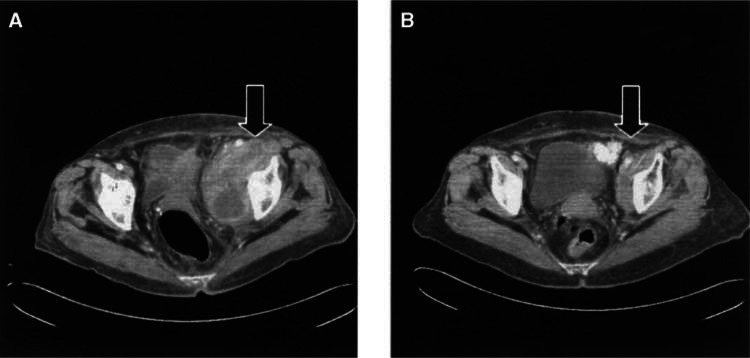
(A) Pelvic CT prior to initiation of low-dose IL-2, in a patient who received adjuvant autologous melanoma vaccine, and had recurrent pelvic LN metastases 12 months later (patient 3). (B) Partial regression of pelvic lymph nodes after 6 months of therapy.
